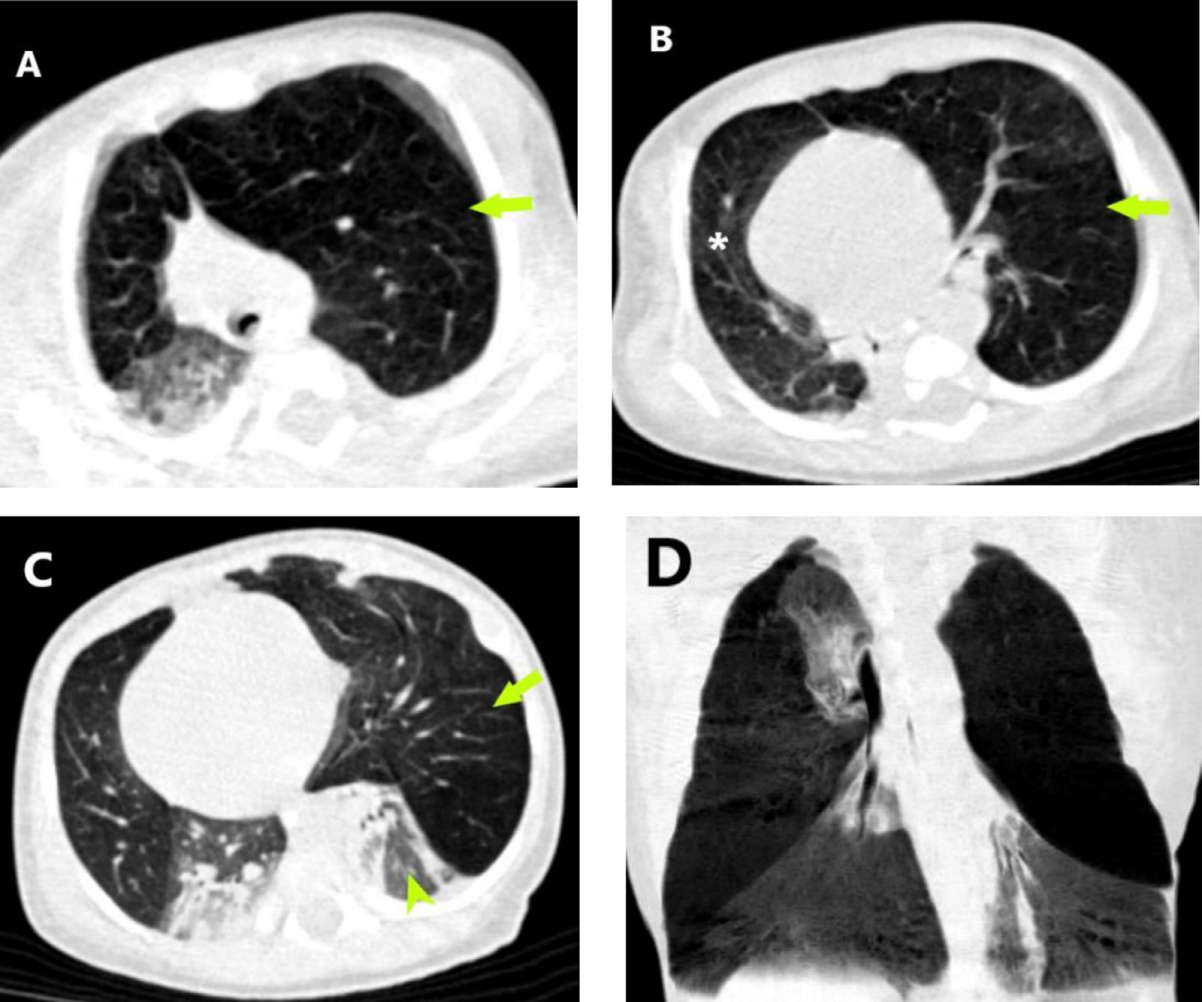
Axial chest CT images in lung window settings at different levels (A through C) demonstrate the presence of marked overinflation of the left upper lobe (arrows) resulting in compressive atelectasis of the left lower lobe (arrowhead) and significant mediastinal shift to the right. Right middle lobe hyperinflation (asterisk) with collapse consolidation of the right upper and lower lobes is also seen (C). Coronal minimal intensity projection (D) better demonstrates the overinflated and collapsed lobes.
